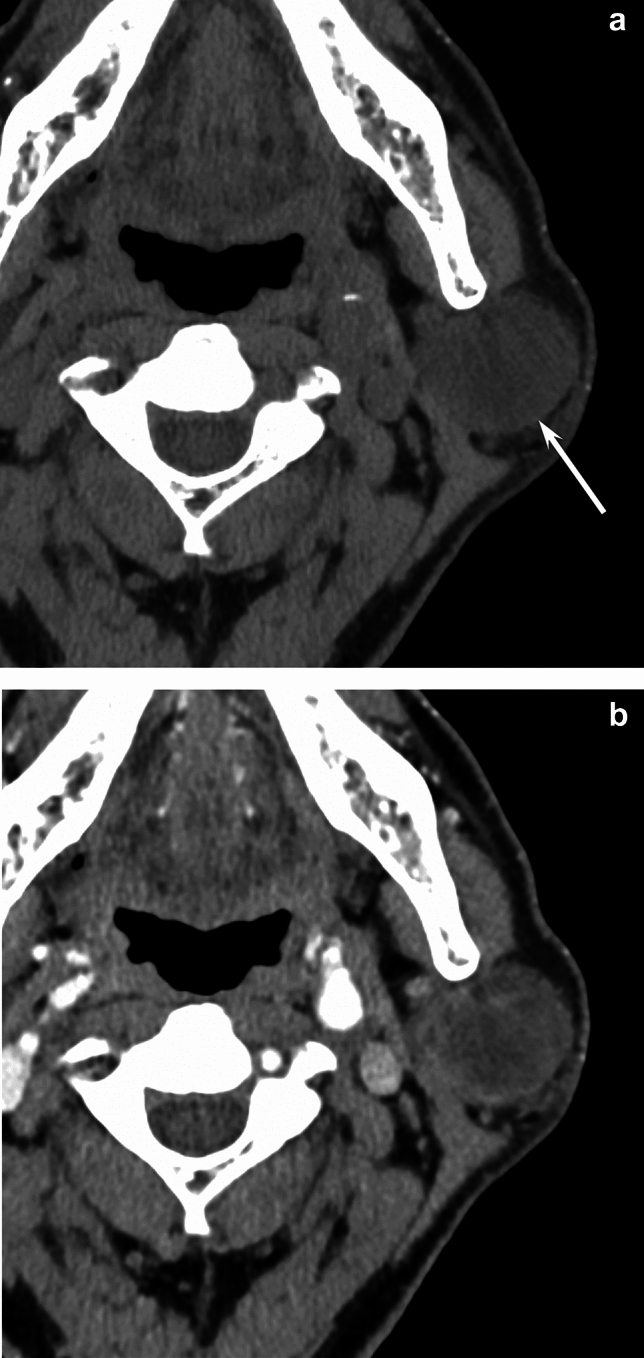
A 72-year-old man with pleomorphic adenoma of the left parotid gland. a Unenhanced CT image shows a hypodense lesion (arrow) (22 HU). b Contrast-enhanced CT image shows mild enhancement (38 HU)
